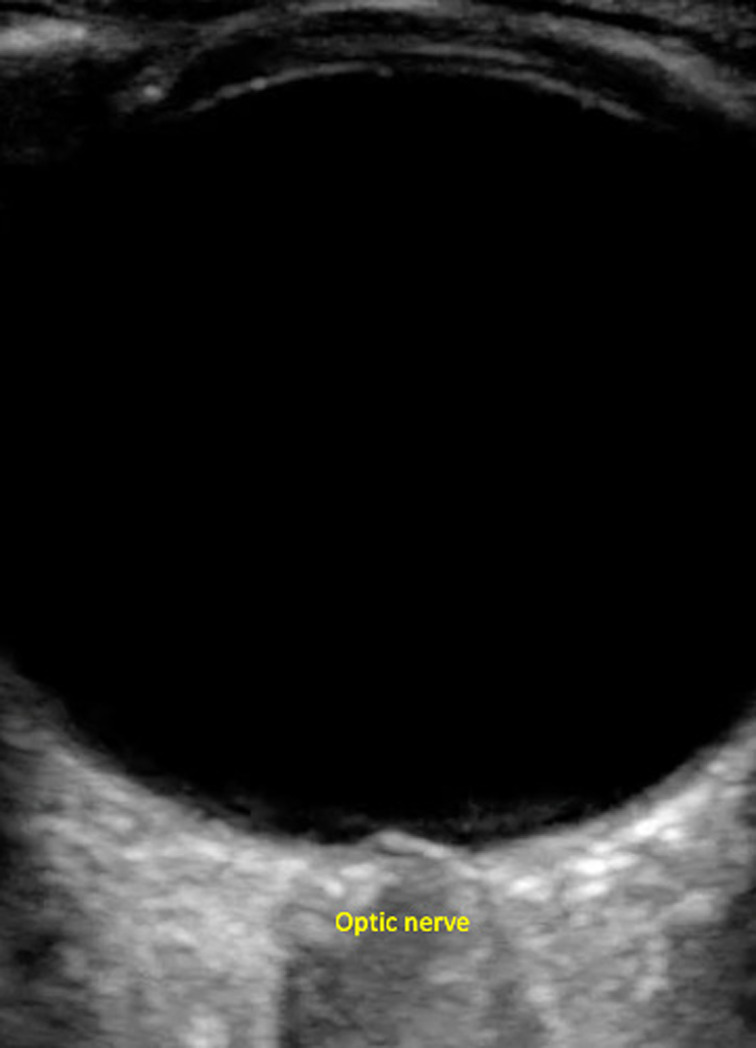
Optic nerve appearance on ultrasound.
